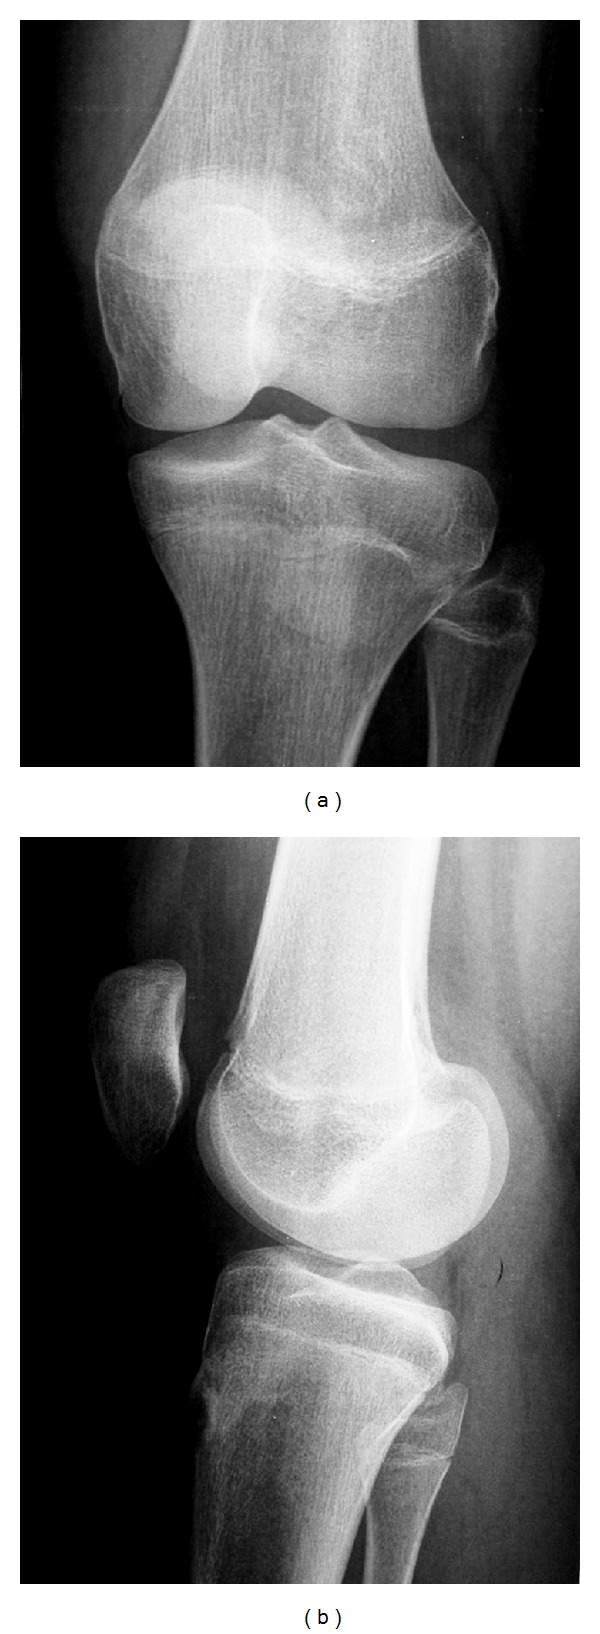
Radiograph of the patient's left knee in anteroposterior (a) and lateral (b) view.
